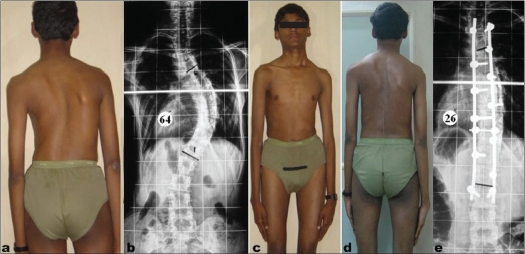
Clinical photograph (a) and anteroposterior radiograph (b) showing typical King type III (T6-L1) curve in Marfan syndrome as the hands have crossed the mid thigh (c). Cobb's angle is 64°. Follow up clinical (d) and radiographic (e) shows correction with Cobb's angle that has been reduced to 26°
